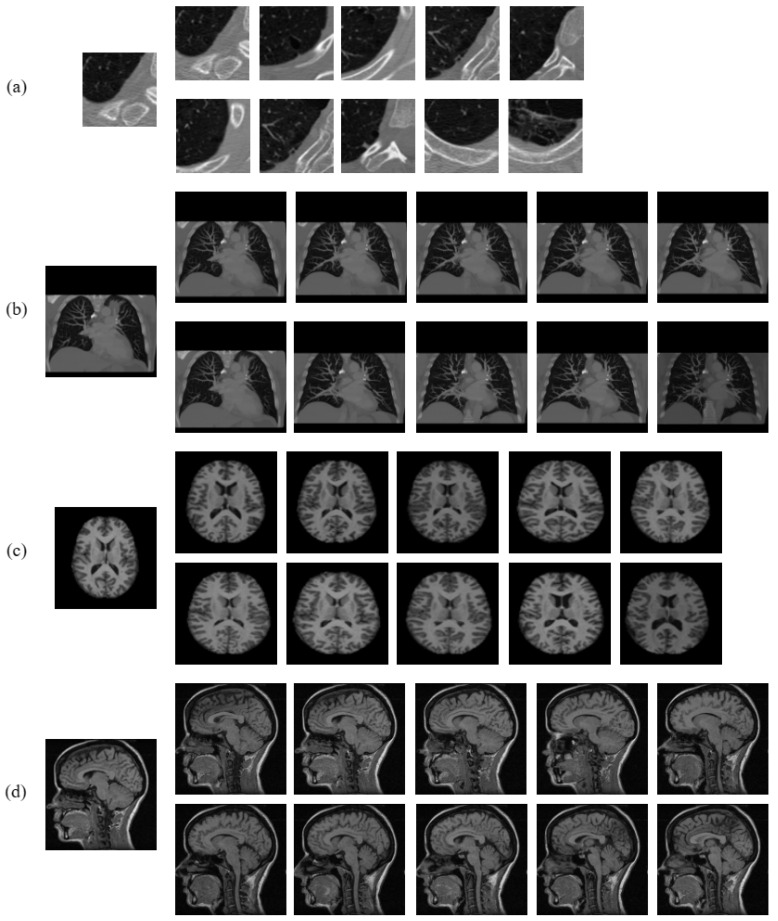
Query results of the proposed method for noise-free query images on (a) Emphysema CT database, (b) NEMA CT database, (c) OASIS MRI database, and (d) NEMA MRI database.
